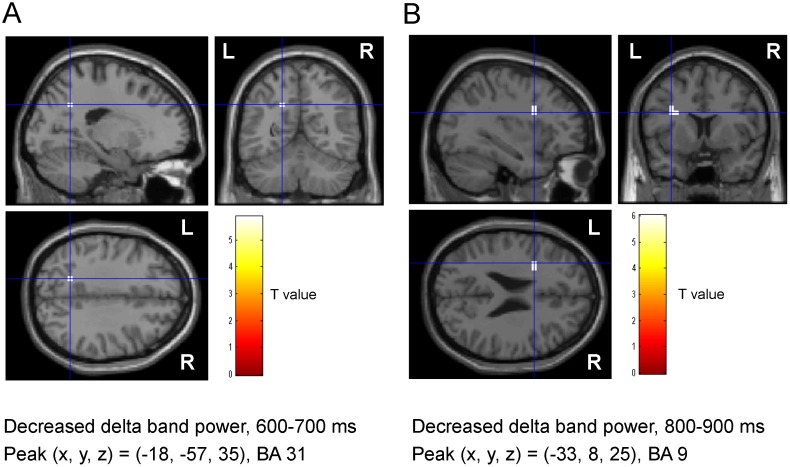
Statistical parametric maps of decreased delta (1–4 Hz) band power in the time windows of 600–700 ms (A) and 800–900 ms (B) after the onset of the execution cue in the evaluation trials relative to the control trials (paired t-test). Statistical parametric maps are superimposed on surface-rendered high-resolution MRI. The extent of the decreased delta band power 600–700 ms after the onset of the execution cue in the brain region extending from Brodmann’s area (BA) 31 (A) and delta band power 800–900 ms after the onset of the execution cue in the brain region extending from BA 9 (B) were greater in evaluation trials than in control trials. The right (R) and left (L) sides are indicated. Random-effect analyses of 13 participants, *P*<0.05, corrected for the entire search volumes (family-wise error rate).
